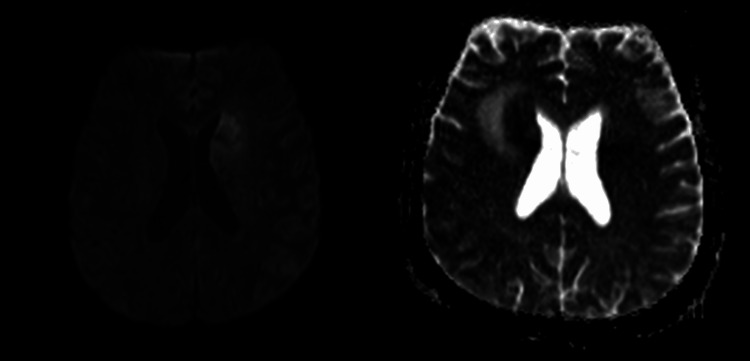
Left frontal lesion demonstrating restricted diffusion on Diffusion‐weighted imaging (DWI)/apparent diffusion coefficient (ADC).
